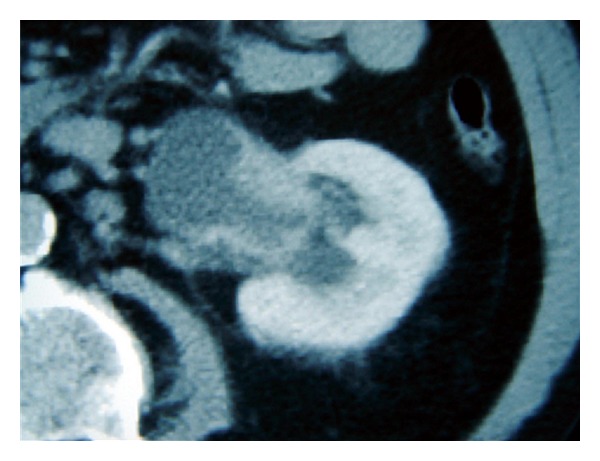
Computed tomography scan showing marked focal thickening of the wall of the inferior aspect of the left renal pelvis extending into the lower pole calyx and into the pelviureteric junction resulting in left hydronephrosis.
